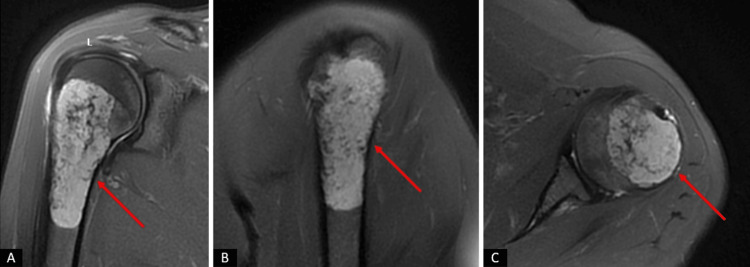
Preoperative magnetic resonance imaging (MRI) scans of the patient of left shoulder. (A) Coronal section MRI images of the patient. (B) Sagittal section MRI images of the patient. (C) Axial section MRI images of the patient. The regions in the proximal humerus with a preliminary diagnosis of enchondroma/low-grade chondrosarcoma are indicated with red arrows.
